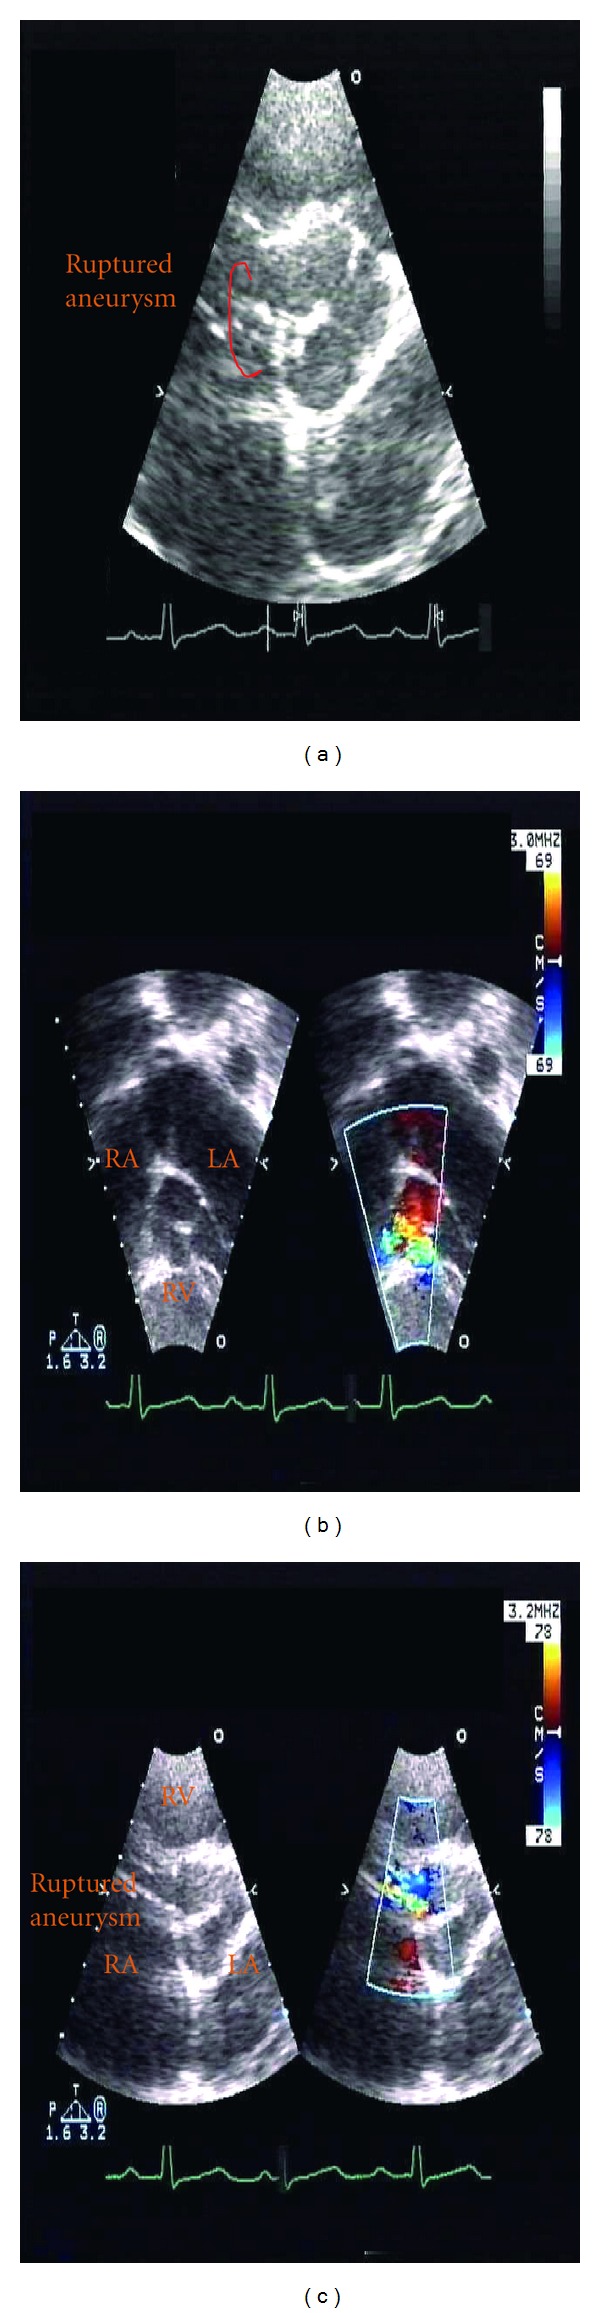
(a) short-axis echo view. Demonstrating fingerlike protrusion evident of ruptured right coronary sinus of Valsalva aneurysm. (b) Subcostal view. (c) Short-axis view of color compared with color flow doppler evidence of aorta to right ventricle fistulous connection.
